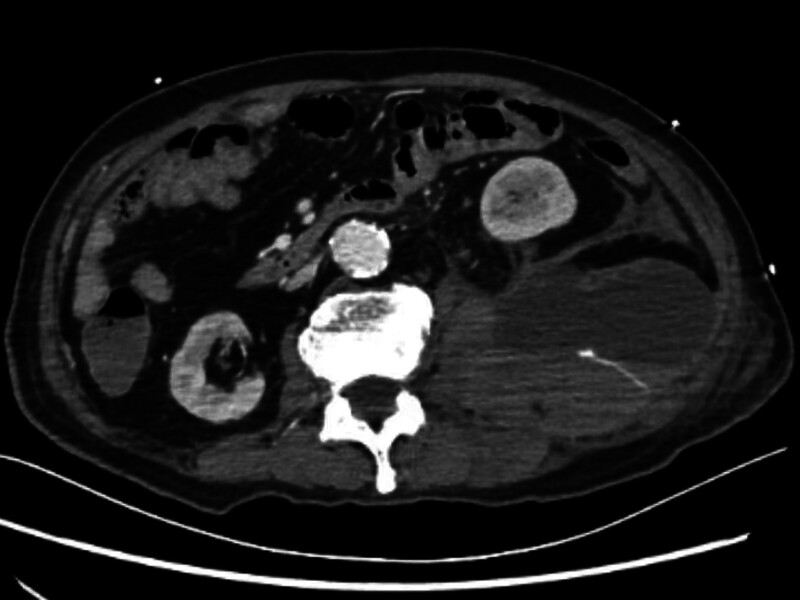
Emergency abdominal CT with contrast showed retroperitoneal hemorrhage.
